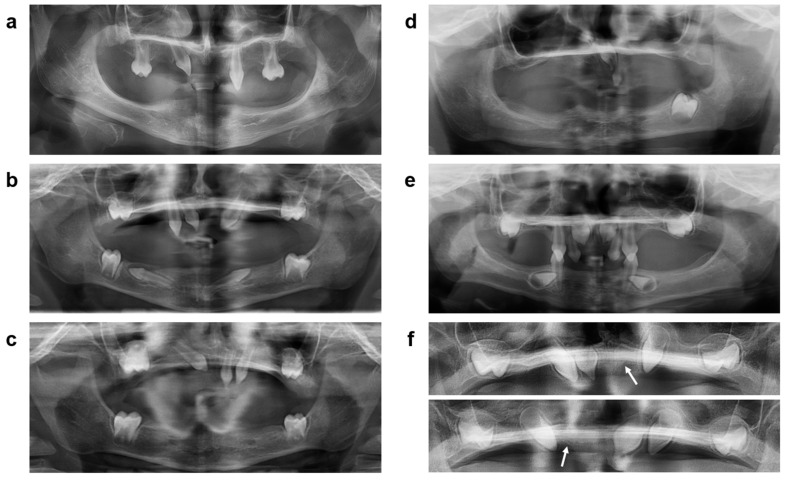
Panoramic dental radiographs of the untreated XLHED-affected brother (a) of subjects P1 and P2 compared with the radiographs of these Fc-EDA-treated subjects at preschool age (b,c) and of the untreated affected brother of P3 (d) compared with the younger sibling 6 years after his prenatal treatment with Fc-EDA (e). Two primary tooth germs ((f), arrowed) that had been detectable in P1 and P2 in early infancy did not undergo complete mineralization and did not erupt.
